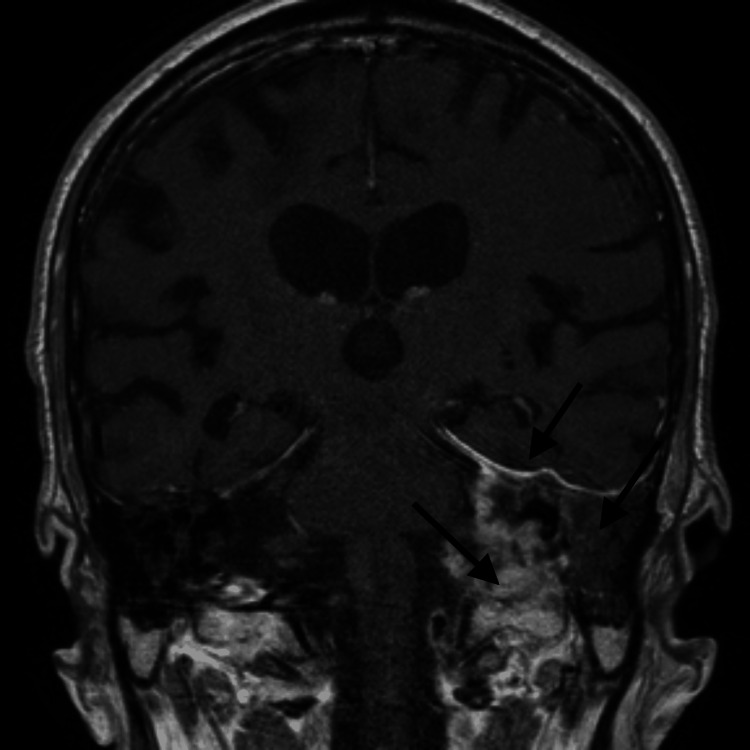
T2-weighted head MRI documenting left otomastoiditis with pachymeningeal inflammation.
